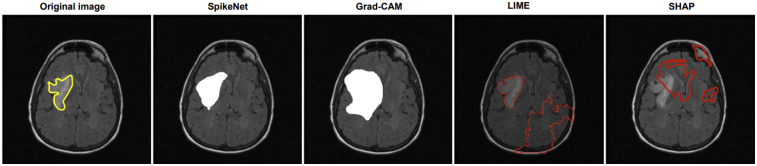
Visual comparison of explanation maps generated for a representative brain MRI slice. The original image includes expert-annotated tumour boundaries (yellow), while the corresponding explanation maps are shown for SpikeNet (white), Grad-CAM (white), LIME (red), and SHAP (red).
